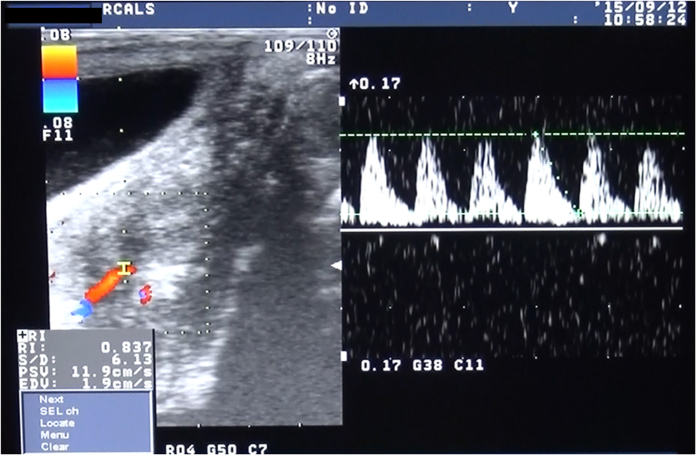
Representative transabdominal ultrasonogram after the transplant. Transabdominal ultrasonography shows good arterial pulsation of right uterine artery 7 weeks after the transplant in case 3.
